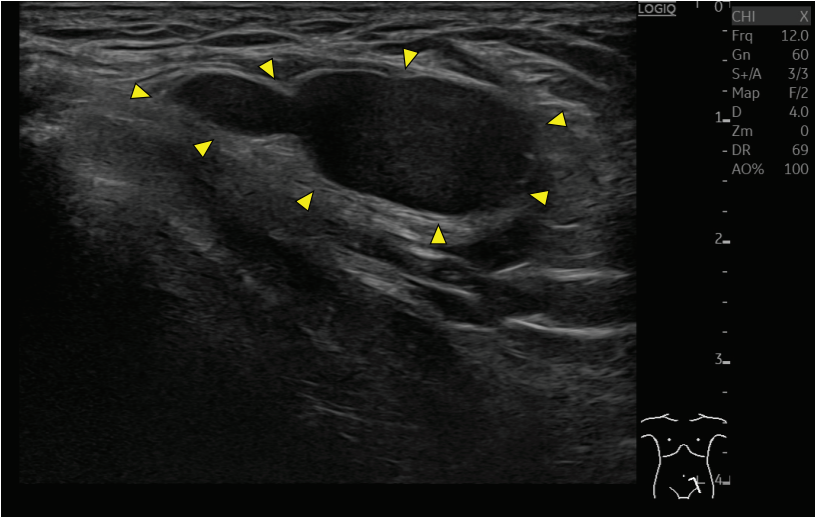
Ultrasonographic findings. A 28 × 11 × 33-mm hypoechoic mass is seen in the subcutaneous fat layer of the left groin (arrowheads). The lesion shows slightly heterogeneous internal echoes, smooth margins, mild posterior acoustic enhancement, and no connection with the epidermis.
